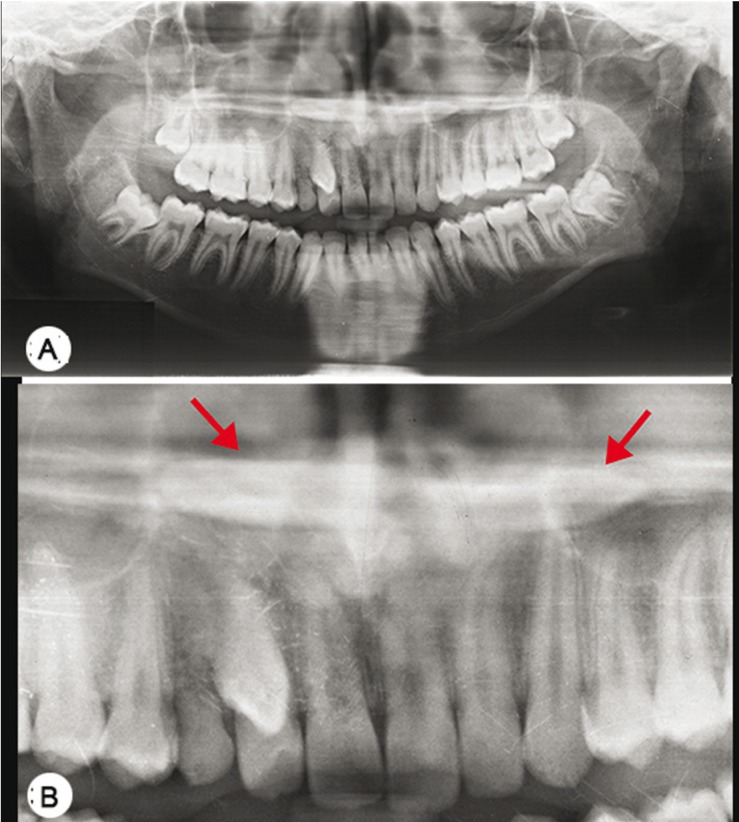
A) Panoramic radiograph showing retained 53 and transposed 13; B) Panoramic radiograph showing shadows of two supernumerary teeth seen above the apices of the anterior sextant.
